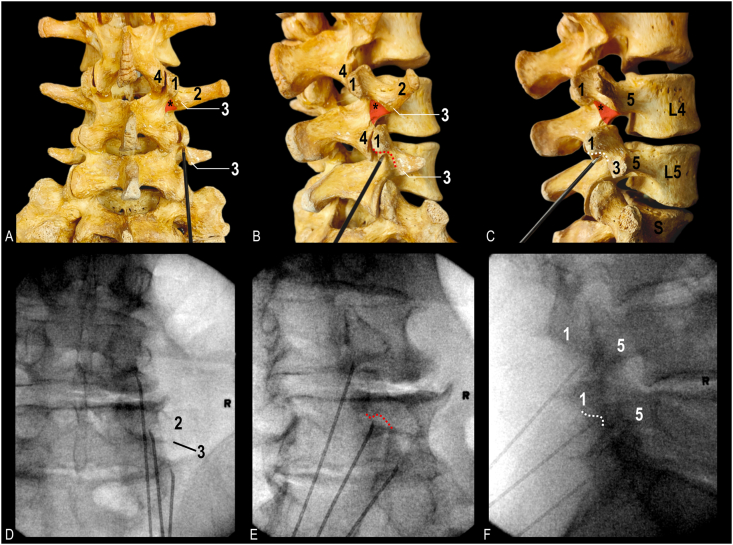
Bony anatomy and fluoroscopic images of cannula placement technique targeting sub-mammillary fossa. A. Posterior view of bony lumbosacral spine with cannula position at sub-mammillary fossa of fifth lumbar vertebra. B. Oblique view. C. Lateral view. D. Radiographic image with cannula targeting the sub-mammillary fossa at the L3 and L4 medial branch level; note cannula targeting L5 medial branch is positioned along the lateral neck. Postero-anterior view. E. Oblique view. F. Lateral view with all 3 cannulae positioned at sub-mammillary fossa. Asterisk indicates second target at superior aspect of sub-mammillary fossa (red triangle); red dotted curve, contour of mamillo-accessory notch seen in oblique view; white dotted curve, contour of mamillo-accessory notch seen in lateral view; 1, mammillary process; 2, transverse process; 3, accessory process; 4, inferior articular process; 5, pedicle; S, sacrum. (For interpretation of the references to colour in this figure legend, the reader is referred to the Web version of this article.)
